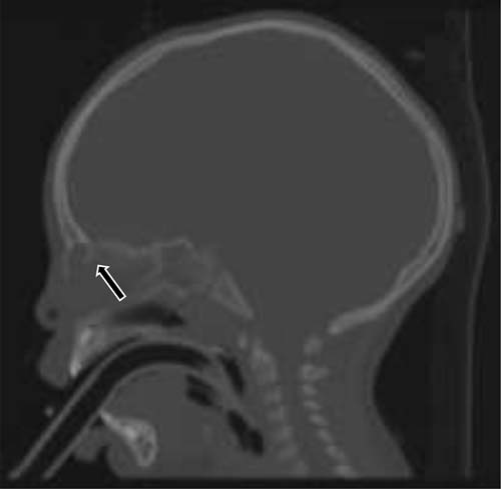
Sagittal cranial CT scan with bone window showing splaying of the nasal bone; a severe osseous defect potentiating extension into the intracranial space.
